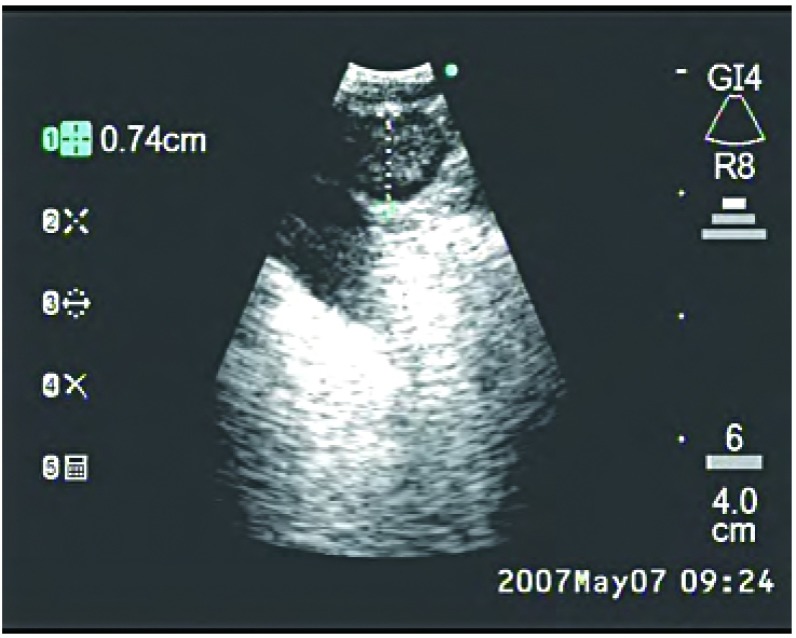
支气管内超声所见肺门淋巴结 Endobronchial ultrasound of hilar node
